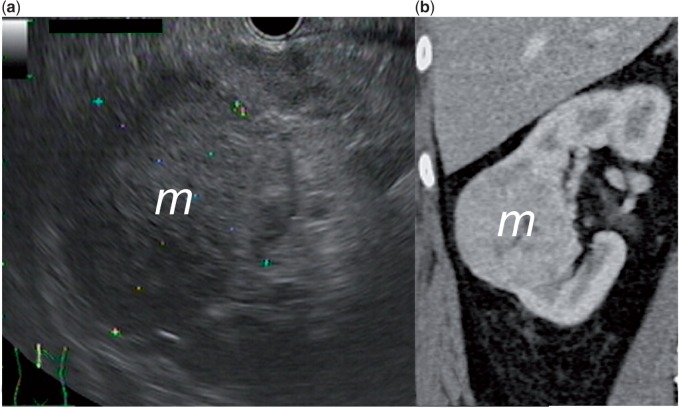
(a) Endoscopic ultrasound image of a 6 cm heterogeneous hypoechoic renal mass. (b) Computer tomography image of the same lesion.
